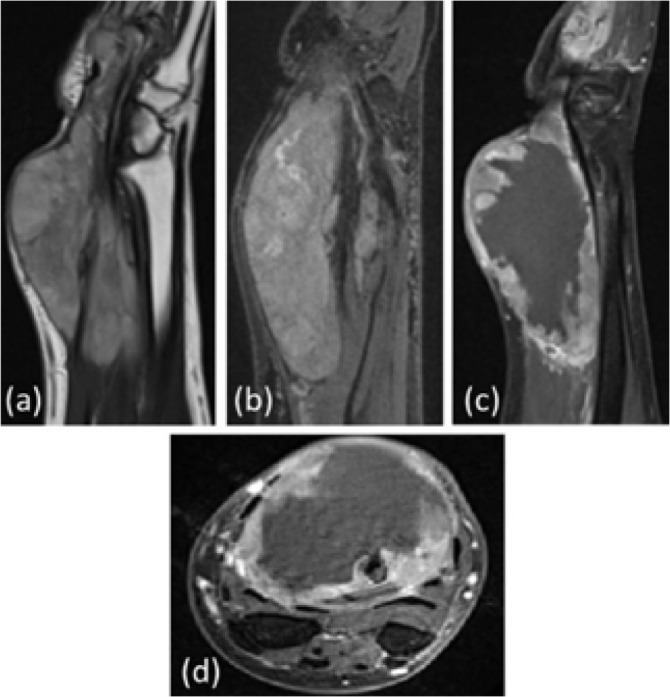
LEFT Wrist MRI. Sagittal T2 WI (a), Coronal T2 fat sat (b), Sagittal T1 fat sat post contrast (c), Axial T1 fat sat with contrast (d). Large volume soft tissue lesion seen within the volar aspect of the left distal forearm measuring 48 x 42 x 98 mm. It demonstrates a heterogeneous signal on the T2 WI sequence with intermediate signal on T1 and demonstrates irregular peripheral enhancement.
